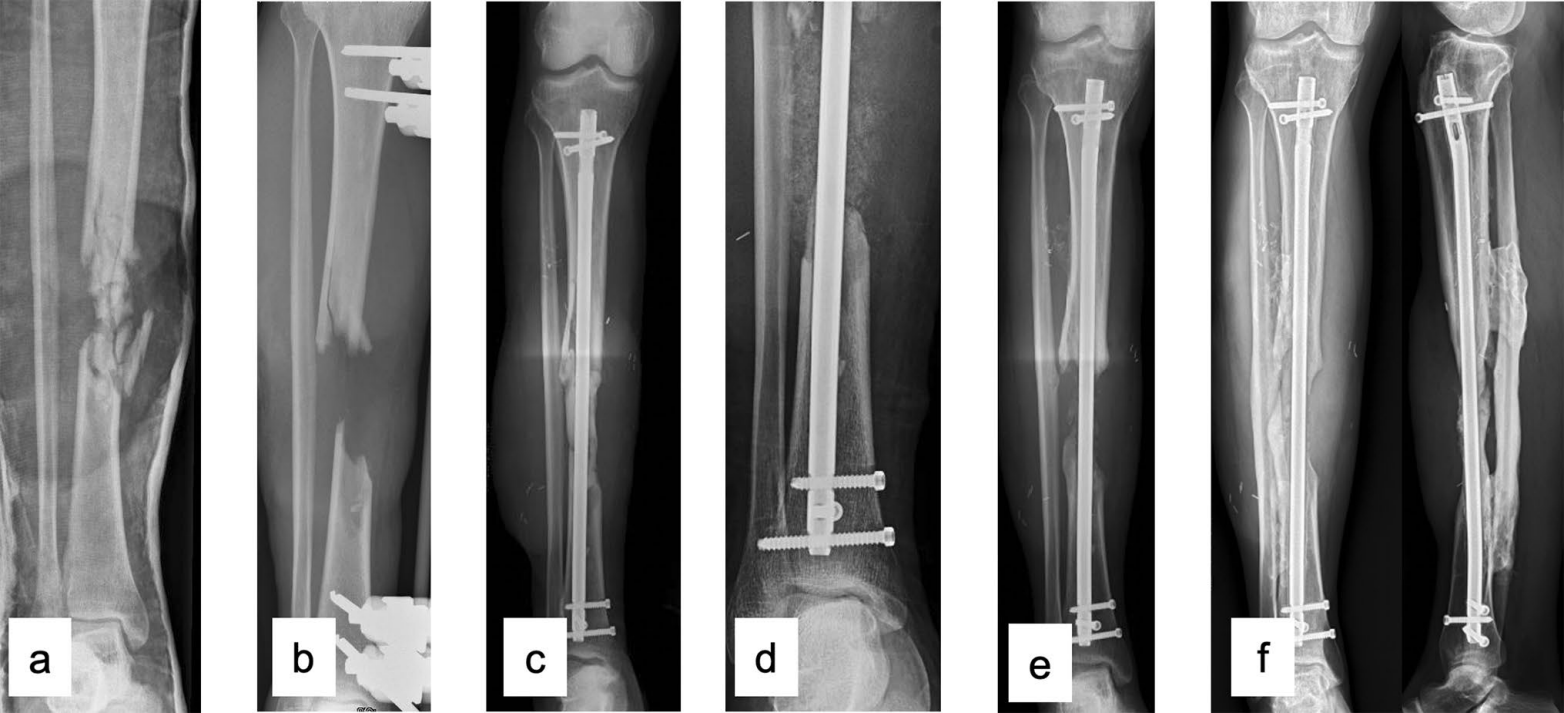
47-year-old male with a Gustilo–Anderson III B shotgun injury, presenting at our department 10 days after injury (a). Debridement, removal of loose bone fragments, and external fixation were performed (b). The patient had positive wound cultures for Enterococcus cloacae and was treated with antibiotics in addition to repeated revision surgery. Twenty-four days after injury, intramedullary nailing of the fracture was performed, and the 9-cm bone defect was filled with a cement spacer in addition to soft tissue closure with a free muscle flap and skin grafting (c). IMT stage 2 was performed 37 days later (d). However, radiographs taken one year after injury showed resorption of the graft (e). Subsequently, a decorticating procedure of the tibia and fibula in addition to autologous bone grafting and implantation of bone morphogenetic protein (BMP2) was performed. Radiographs obtained at follow-up 69 months after the initial treatment show a tibiofibular bone-bridge both proximal and distal to the bone defect (f)
